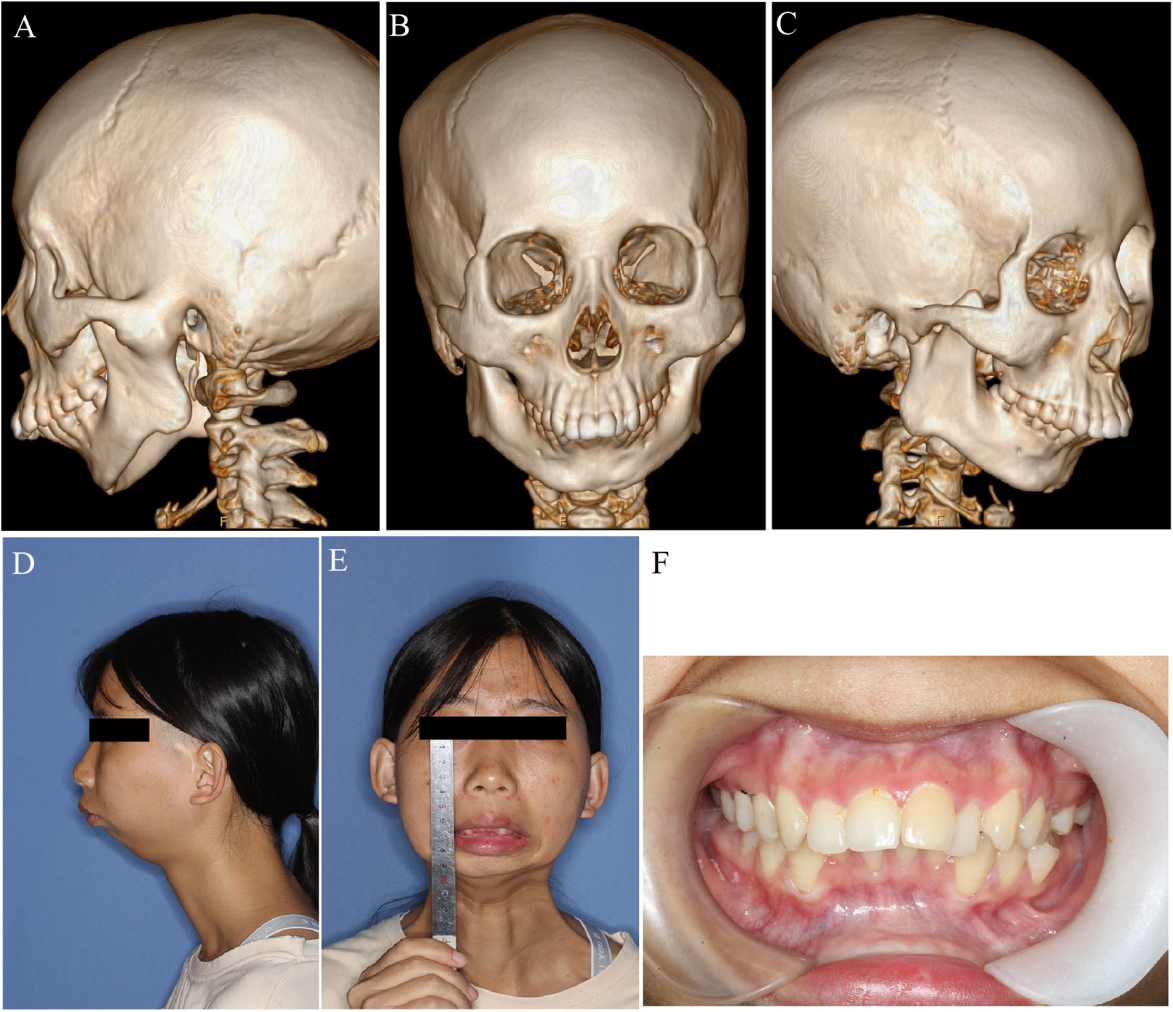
Preoperative profile of a 21 years old female patient with L TMJ ankylosis. (A) Left lateral CT view showing bony ankylosis; (B) Frontal CT view; (C) Right lateral CT view; (D) Left lateral view of facial profile; (E) Frontal view showing complete absence of mouth opening; (F) Intraoral image demonstrating complete trismus with no interincisal opening.
